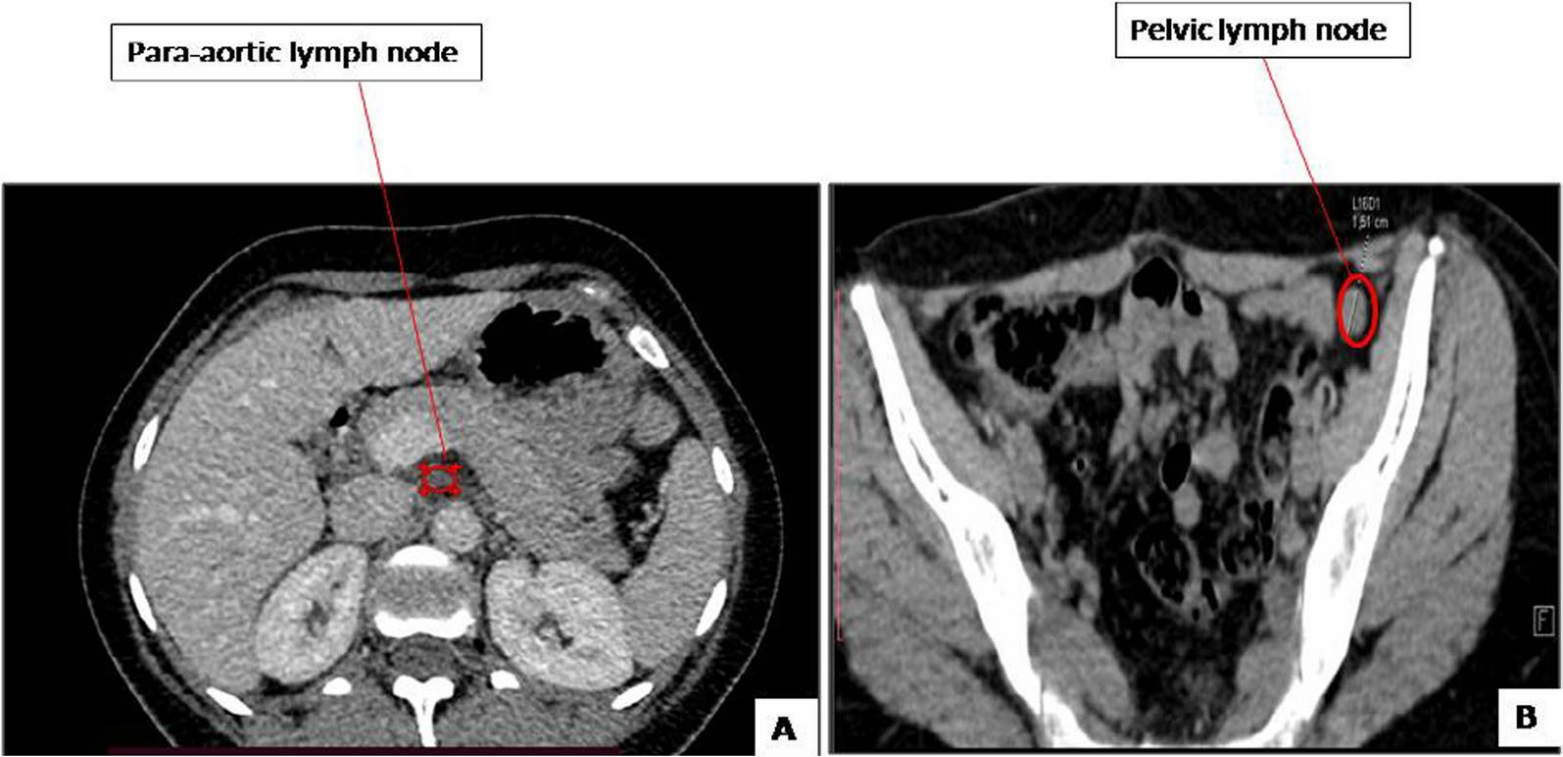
A Computed tomography scan showing paraaortic lymph nod (appointed with red arrow) e measuring 17 × 12 mm and B left pelvic lymph node (appointed with red arrow) measuring 16 × 5 × 10 mm
